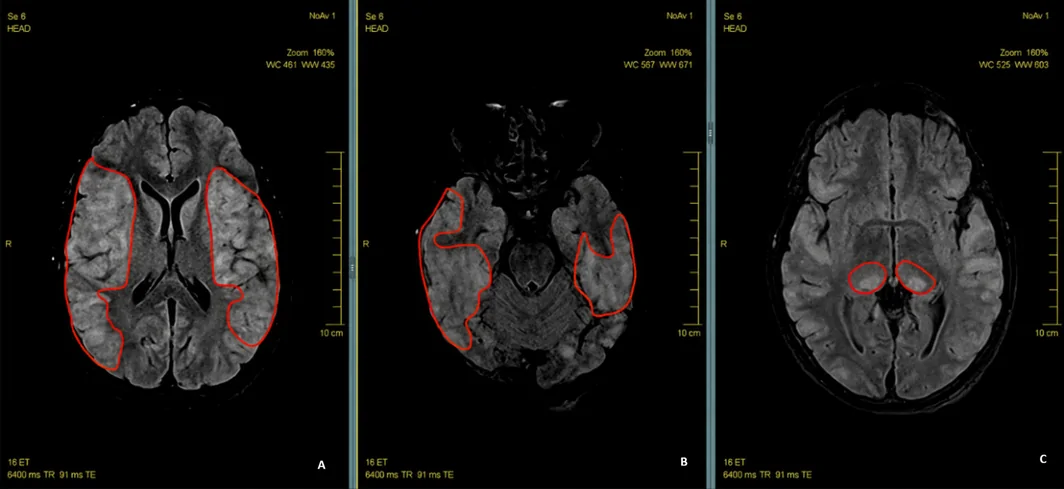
Axial T2/FLAIR MRI sequence demonstrating bilateral temporal and thalamic hyperintensities consistent with autoimmune encephalitis. (A) Bilateral cortical and subcortical temporal lobe hyperintensities. (B) Predominant Bilateral temporal lobe involvement. (C) Bilateral thalamic hyperintensities.
